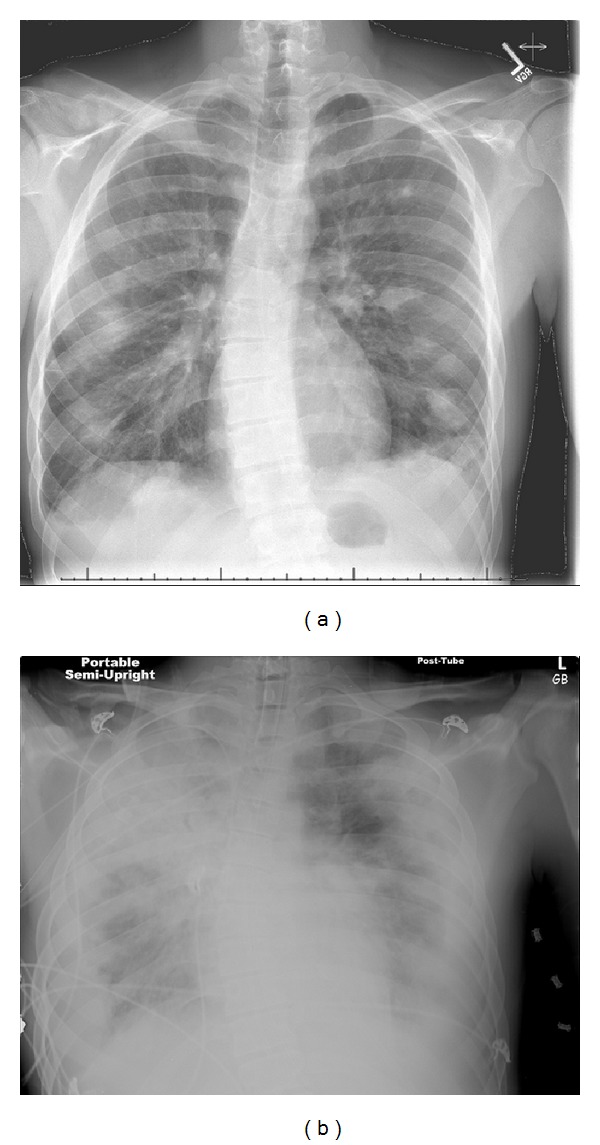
(a) Case 1 CXR early pulmonary nodules and infiltrates (cavitating on CT). (b) Case 1 CXR developed into dense bilateral infiltrates and ARDS.
